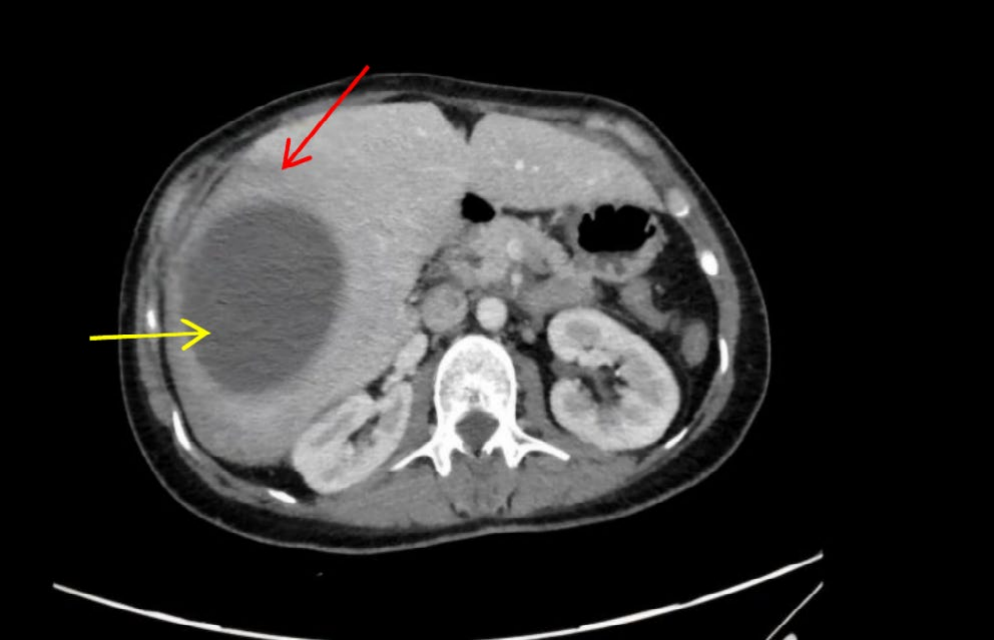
CT scan after the operation and the evacuation of the hematoma.
